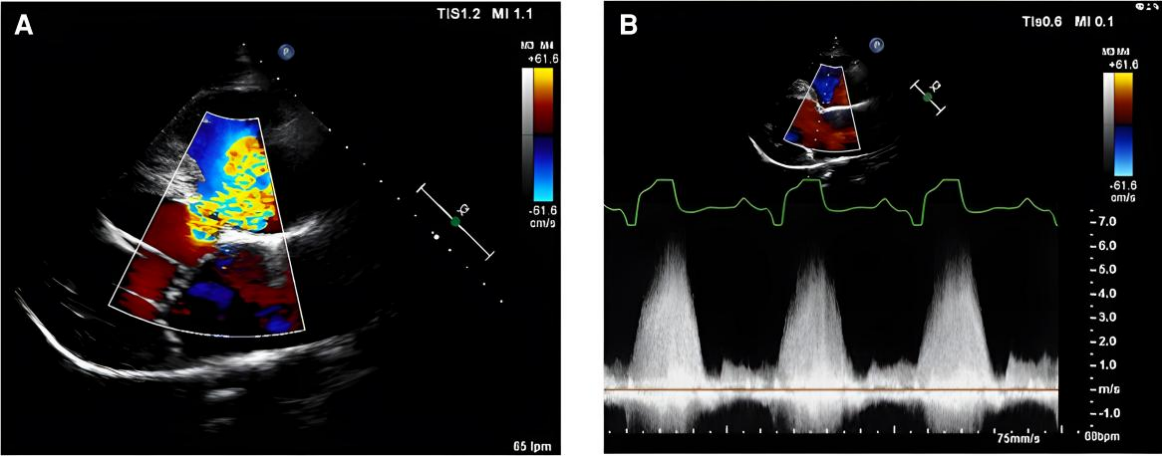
Transthoracic echocardiogram image of subpulmonary ventricular septal defect (VSD). (*A*) Parasternal long-axis plane with colour Doppler showing interventricular shunt with unidirectional flow from the subaortic right ventricle to the subpulmonary left ventricle. (*B*) Continuous Doppler flow of the VSD with a maximum velocity of 6 m/s.
